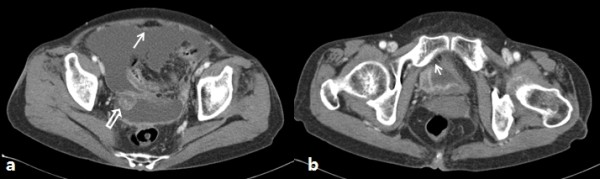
Postoperative computed tomography. a. Computed tomography revealed large amount of ascites with enhancing peritoneal thickening (arrow) and peripheral enhancement of the right adnexa (empty arrow). b. Bladder showed diffuse nodular enhancing wall thickening and focal defect in the anterior wall, suggesting perforation (arrow).
